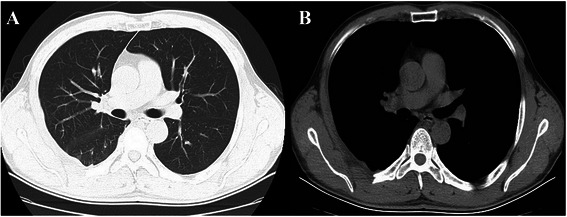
Follow-up chest computed tomography scan. No residual tumor or recurrence was found in the lungs (a) or chest wall (b)
